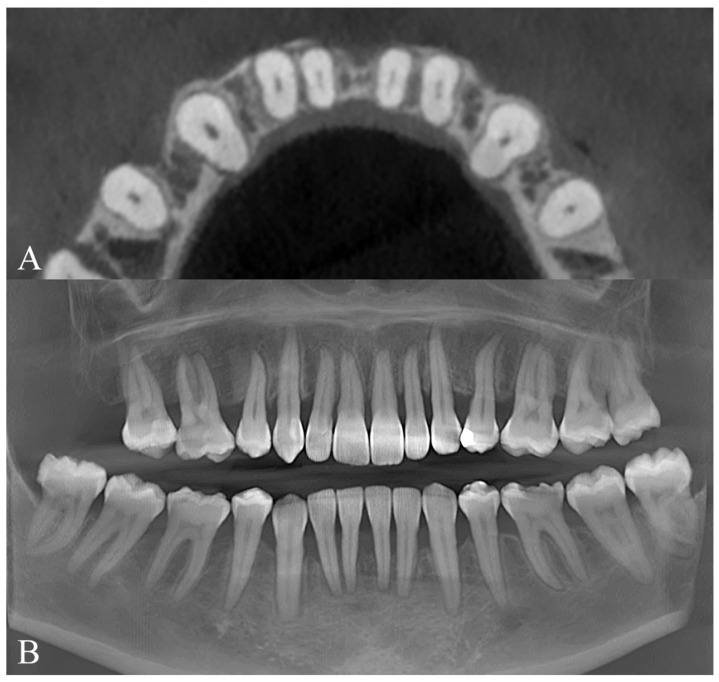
Final radiographic records of Clinical Case 1. (A) CBCT sections of the mandibular anterior region. (B) Panoramic radiograph reconstructed from the CBCT.
